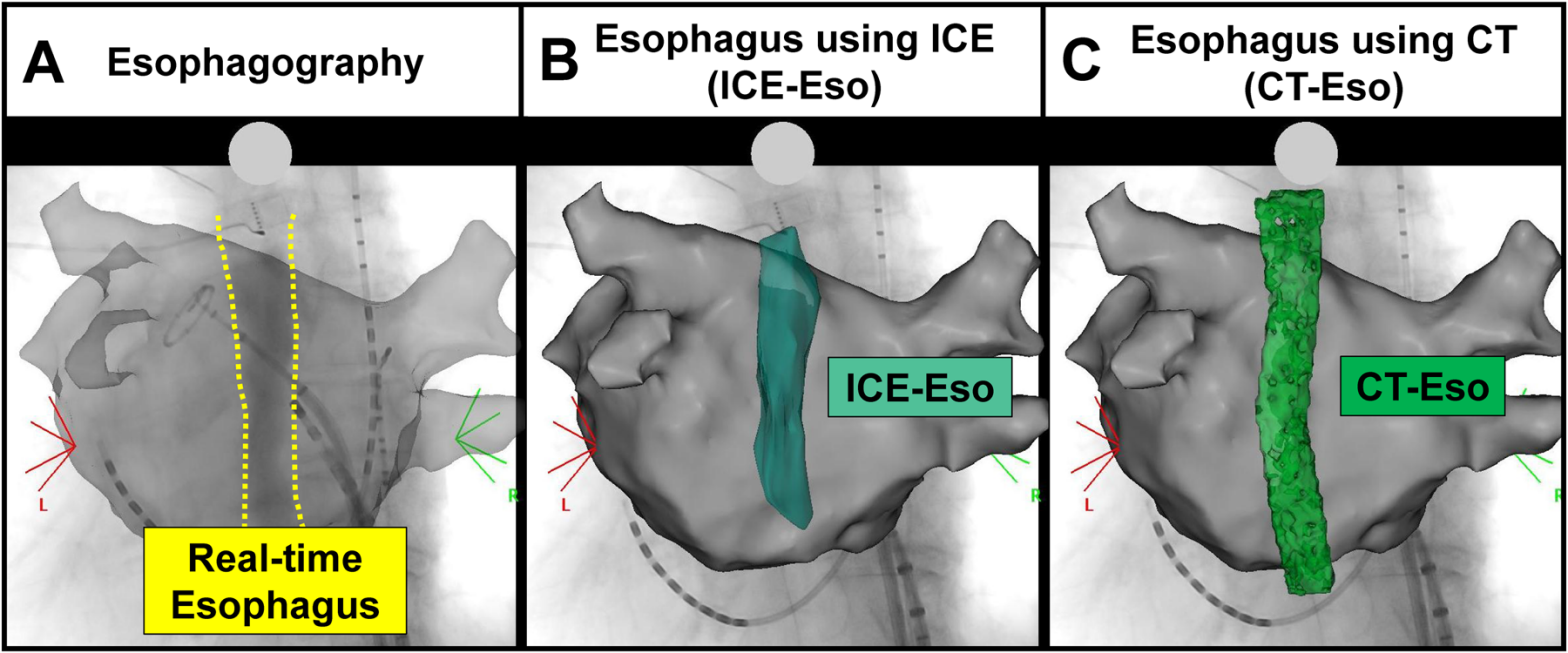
Combination Multimodality Imaging on Three‐Dimensional Mapping System. Multimodality imaging combined with fluoroscopy and CARTO3 system using CARTOUNIVIU module. (Panel A: Contrast esophagography; Panel B: Intracardiac echocardiography; Panel C: Computed tomography.)
